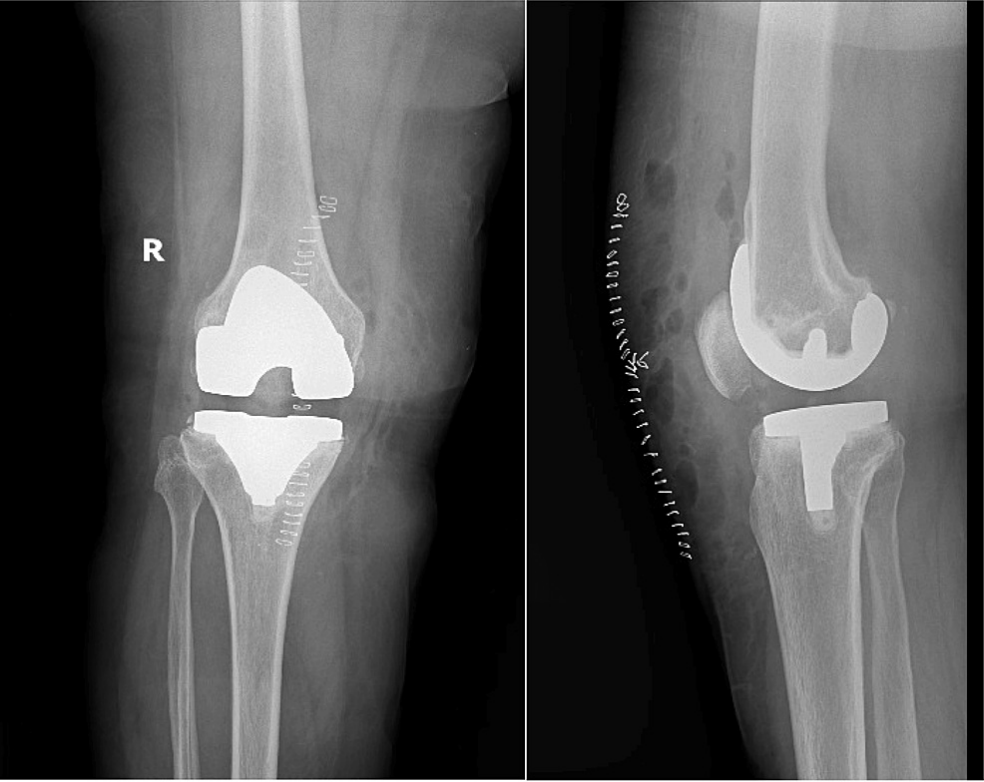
Postoperative radiograph of the right knee in the supine position
